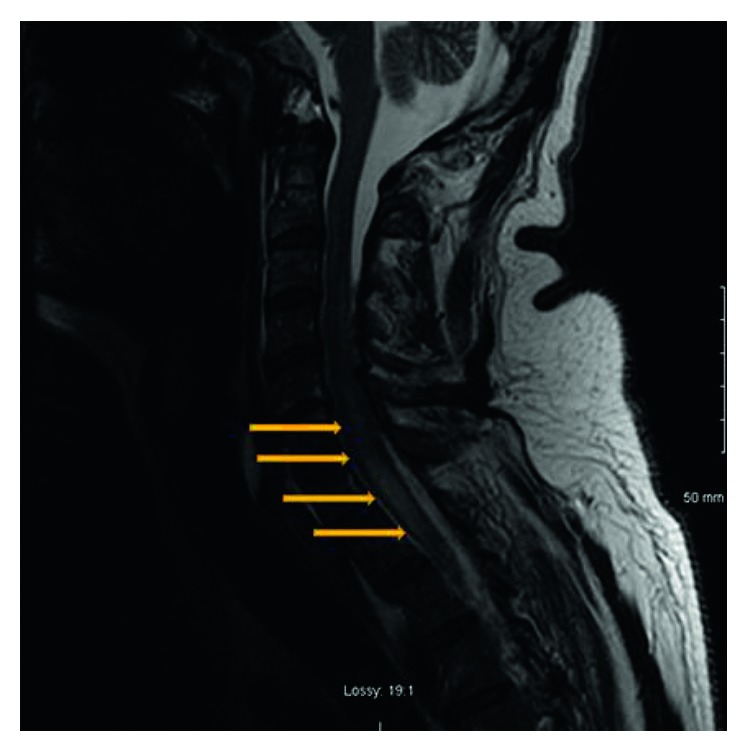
A sagittal T-weighted MRI of the C-spine of the patient on May 22, 2017, after the antifungal treatment. The arrows point to the spinal cord lesion due to coccidioidal infection; however, the size of the lesion has decreased significantly in comparison to Figure 1.
